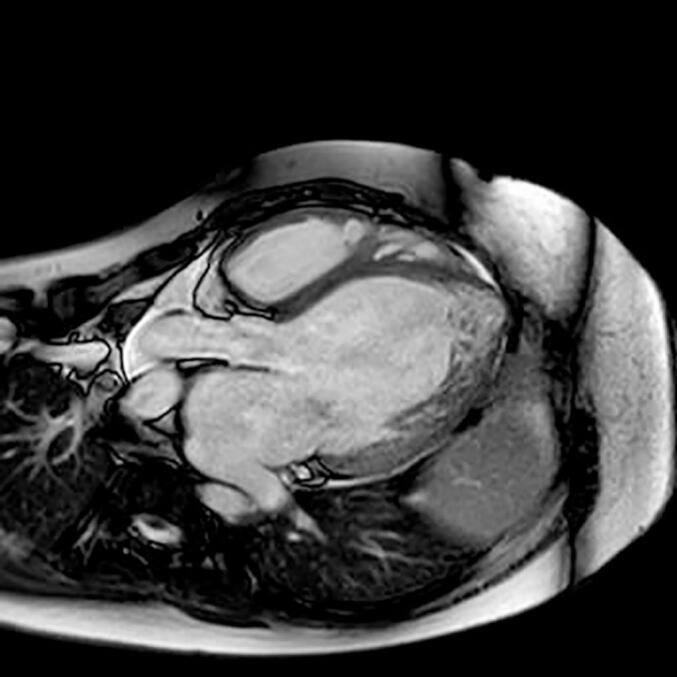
Cardiac MRI performed before the LVAD (HM3)-implantation showing markedly dilated left chamber with excessive trabeculation.
